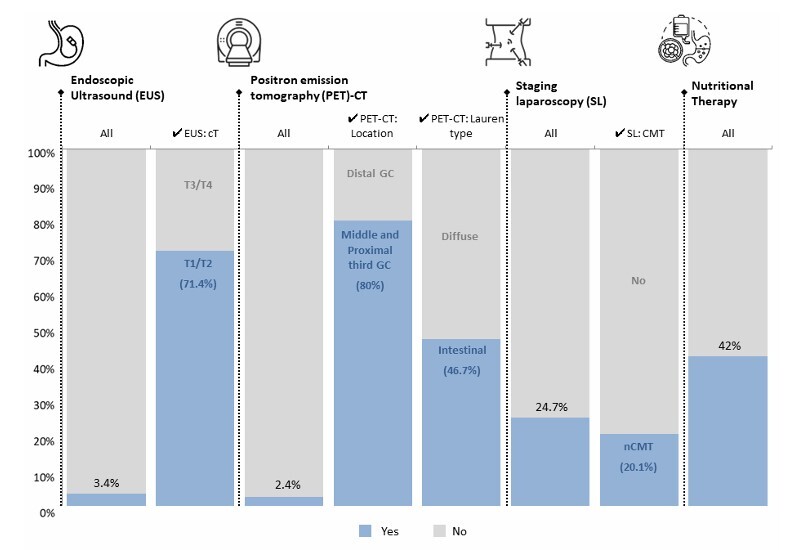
Frequency of endoscopic ultrasound, positron emission tomography-computed tomography, staging laparoscopy, and preoperative nutritional therapy. EUS: endoscopic ultrasound; PET-CT: positron emission tomography-computed tomography; SL: staging laparoscopy; CMT: chemotherapy.
